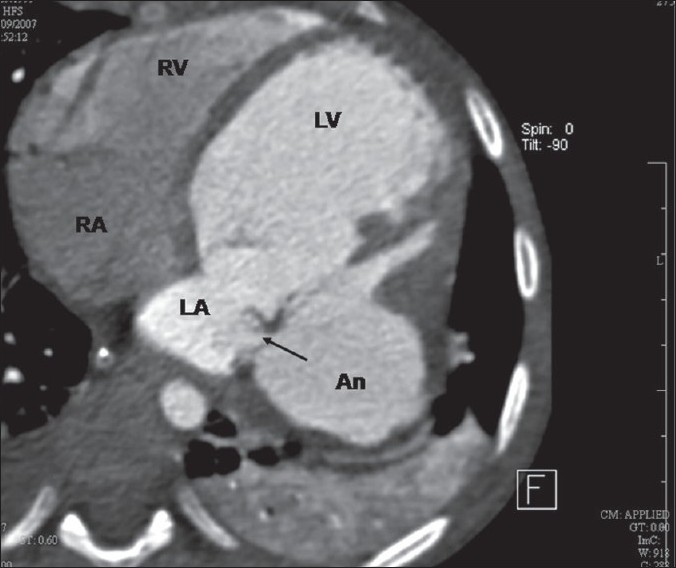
A CT scan shows the precise extent of the aneurysm and its relation to the neighboring structures. Arrow points towards the site of rupture into the left atrium
